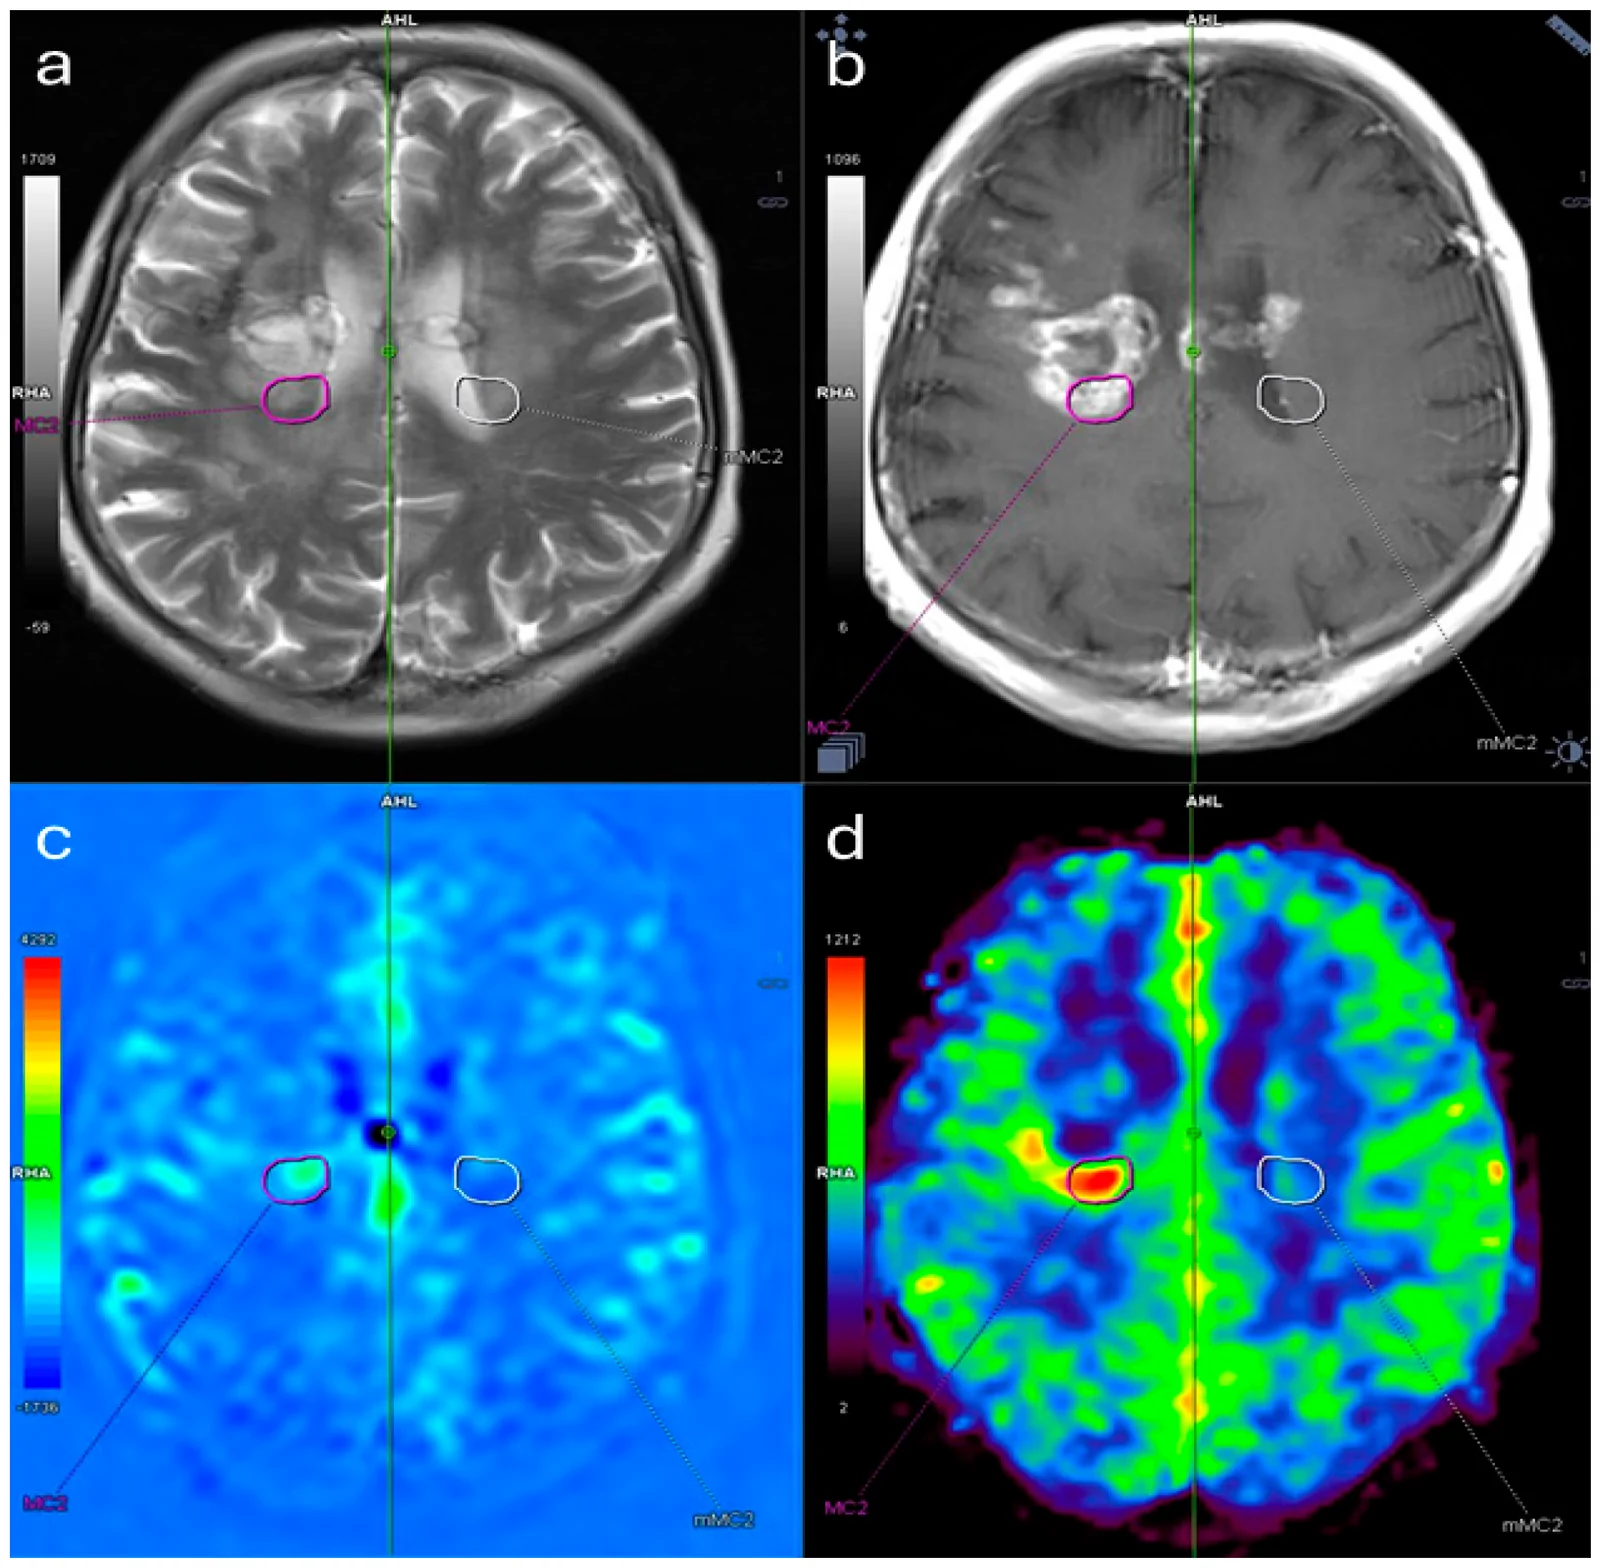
Tumor recurrence in a 64-year-old female patient with glioblastoma. Axial T2-weighted (a) and post-contrast T1-weighted (b) images show a lesion adjacent to the postoperative cavity. Arterial spin labeling (ASL) (c) and dynamic susceptibility contrast (DSC) (d) perfusion images demonstrate increased perfusion, consistent with tumor recurrence. Colored outlines indicate the lesion region of interest, and white outlines indicate the corresponding contralateral reference region used for comparison.
